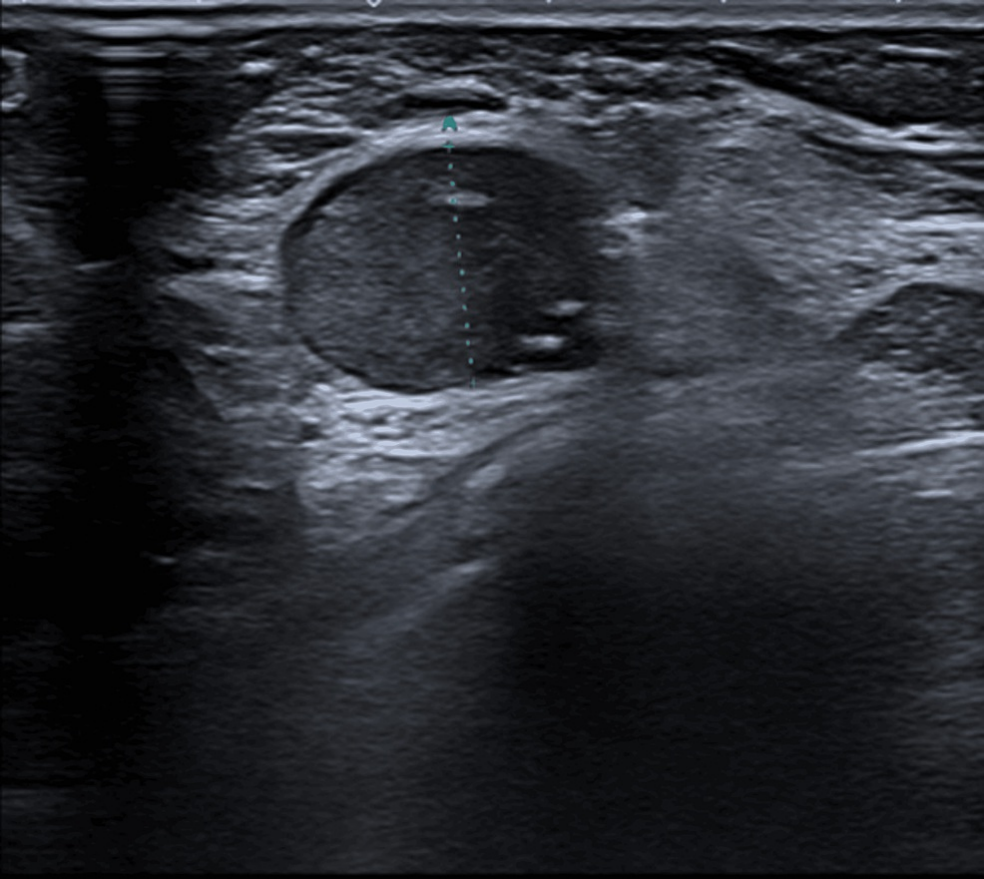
Ultrasound of the left axilla. The image shows one of the suspicious lymph nodes in the left axilla. Fine-needle aspiration cytology of one of these lymph nodes was performed.
